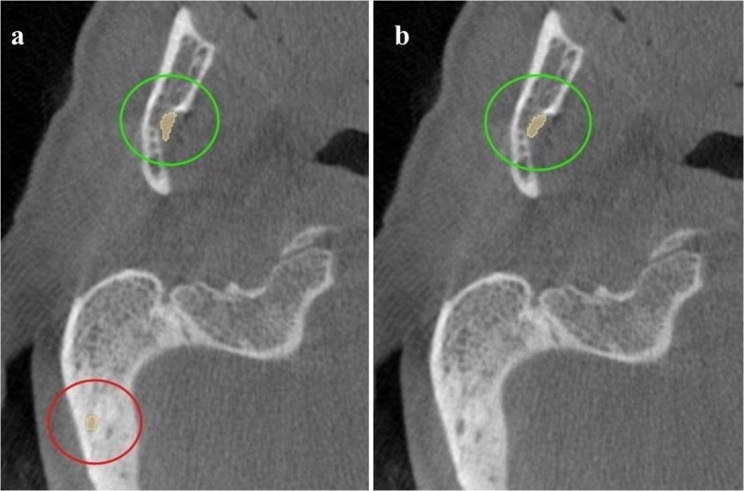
Axial CBCT slices showing post-processing effects on segmentation: (a) initial prediction with an extraneous component (red circle); (b) final output after removal, retaining only the mandibular canal (green circle)
